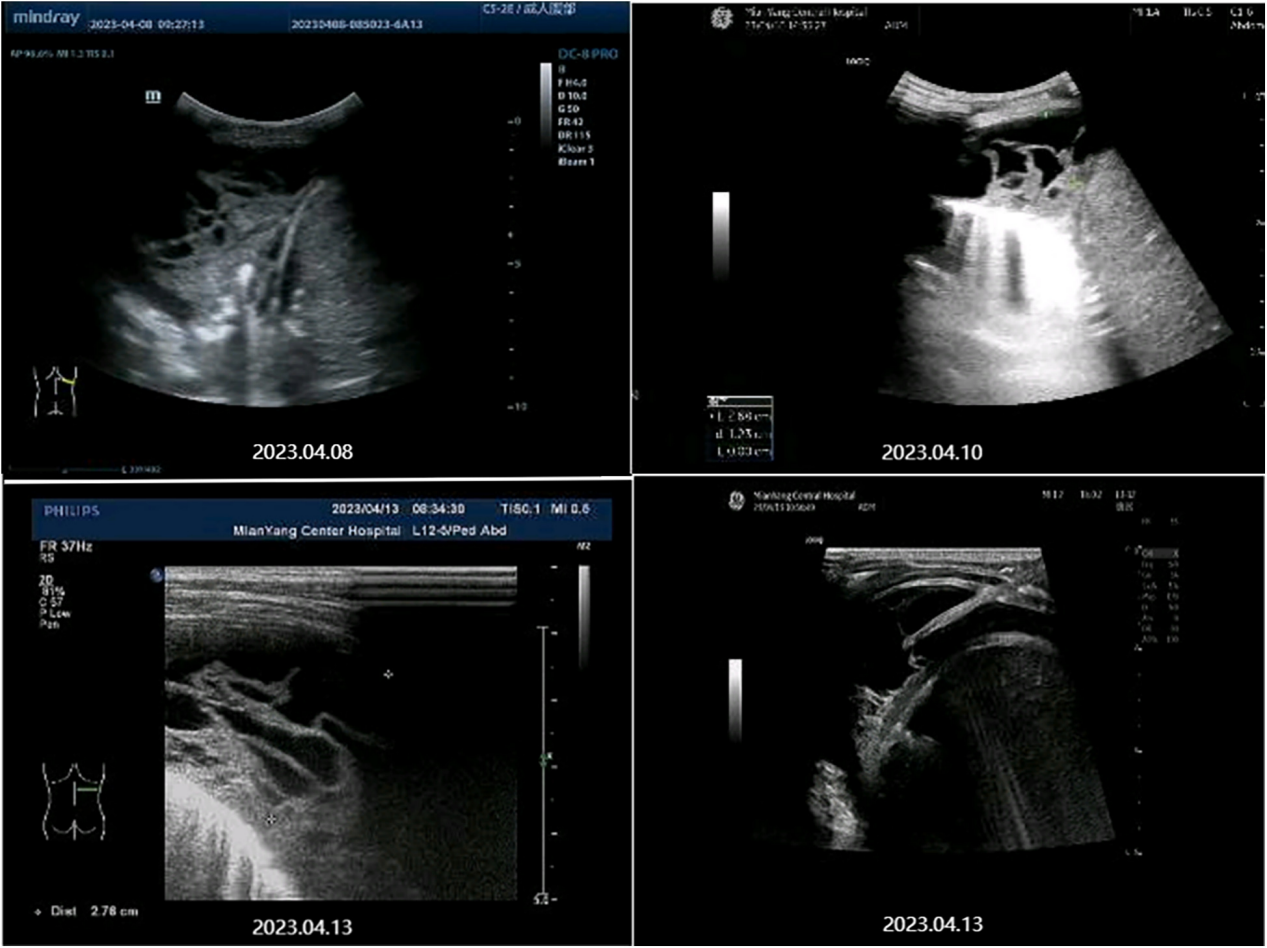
Chest color ultrasound images before treatment.
